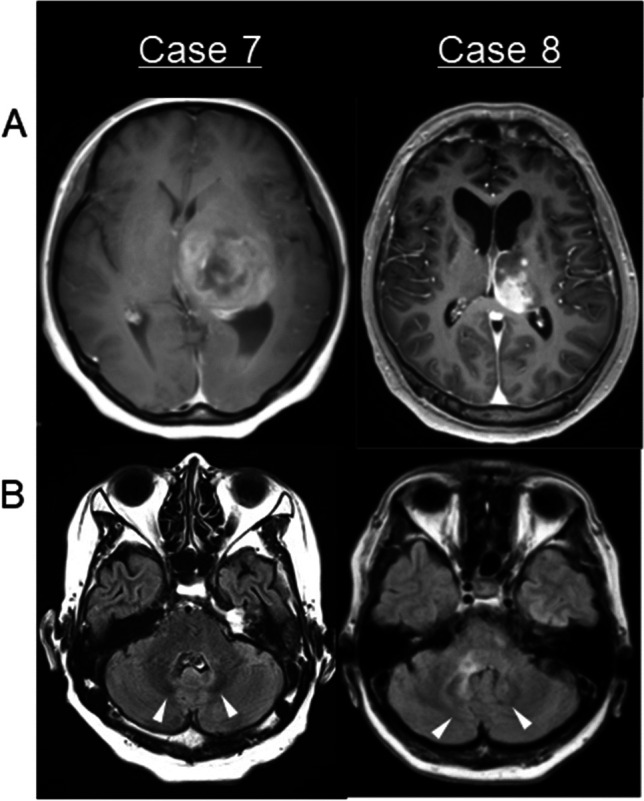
Cases of thalamic diffuse midline glioma, H3 K27-altered (case 7) and glioblastoma (case 8) with recurrence in the cerebellar DN. A Gd-T1WI at the initial presentation showing the enhanced area in the contralateral DN. B FLAIR 6 and 7 months after tumor resection showing recurrence at the DN and its hilus (arrowhead)
